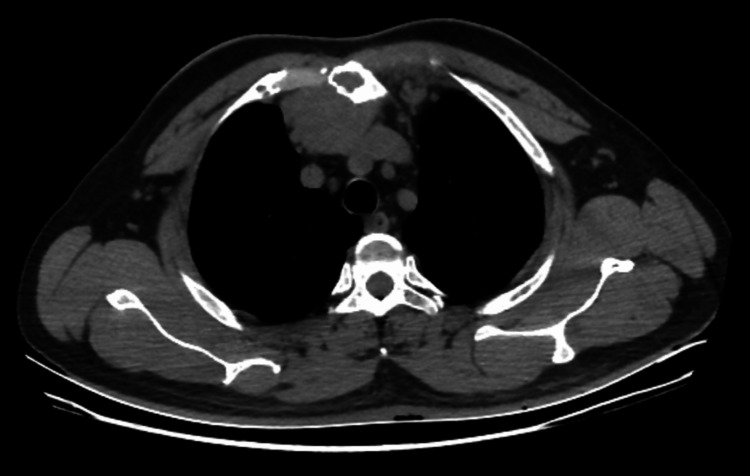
Contrast-enhanced computed tomography (CECT) of the chest A well-defined, heterogeneously enhanced soft tissue mass on the right side of the manubrium sterni.
